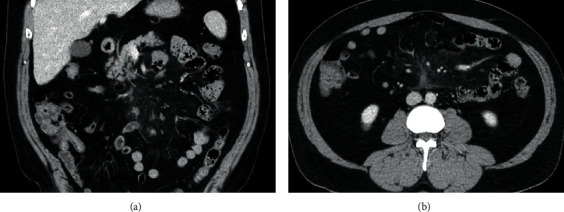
CT abdomen w/contrast: there is moderate “misty” infiltration of the root of the small bowel mesentery (arrows), where an increased number of subcentimeter normal-size mesenteric lymph nodes are also identified. (a) Coronal view. (b) Axial view.
